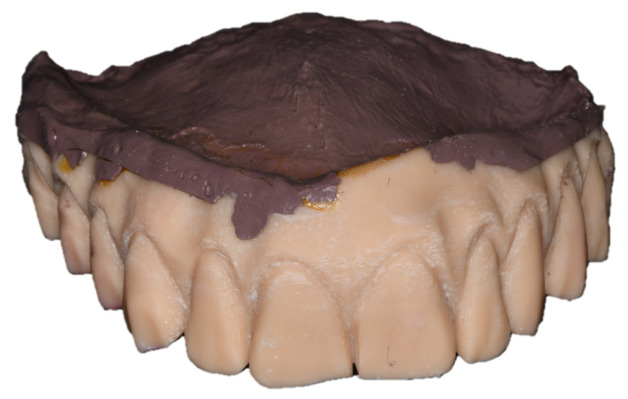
Duplication of the clinically validated prosthetic prototype and fabrication of the radiographic template for the double-scan CBCT protocol.
